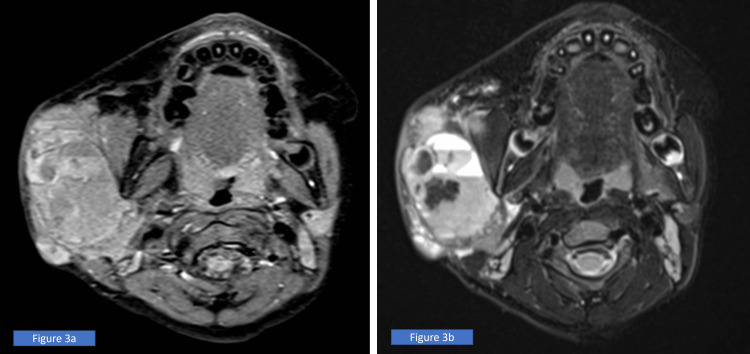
(a) Contrasted MRI T1 and (b) contrasted MRI T2 with an axial view at the level of the angle of the mandible showed a lesion that is confined in the superficial and deep lobe of the parotid with multiple fluid levels and presence of acute and subacute bleeding. There is contrast enhancement predominantly at the inferior portion and septa, with mass effect. No parapharyngeal space, postauricular, or intramuscular extension.
